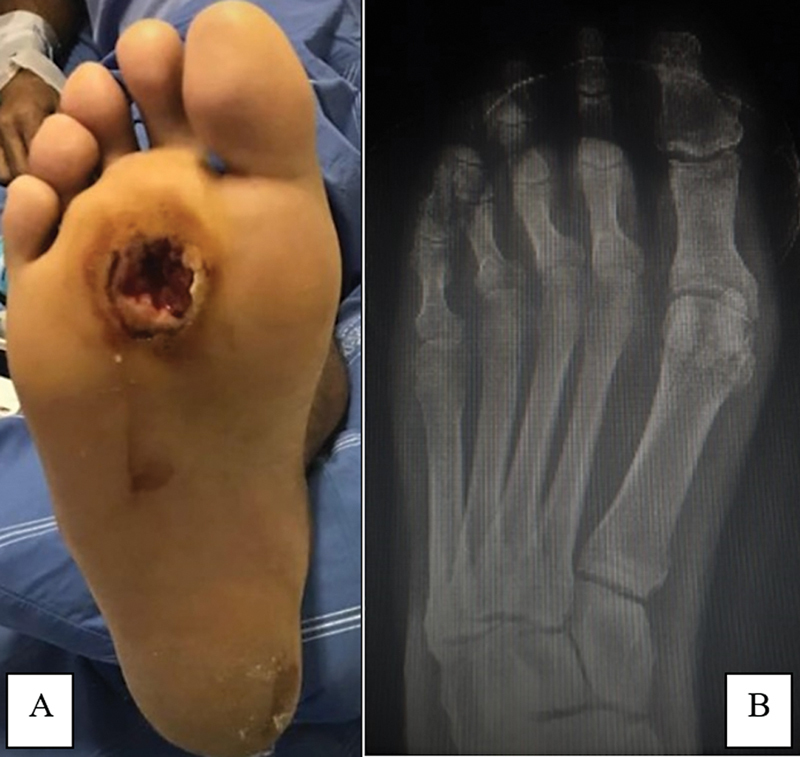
Clinical and radiographic appearance of the right foot on the first visit. (
